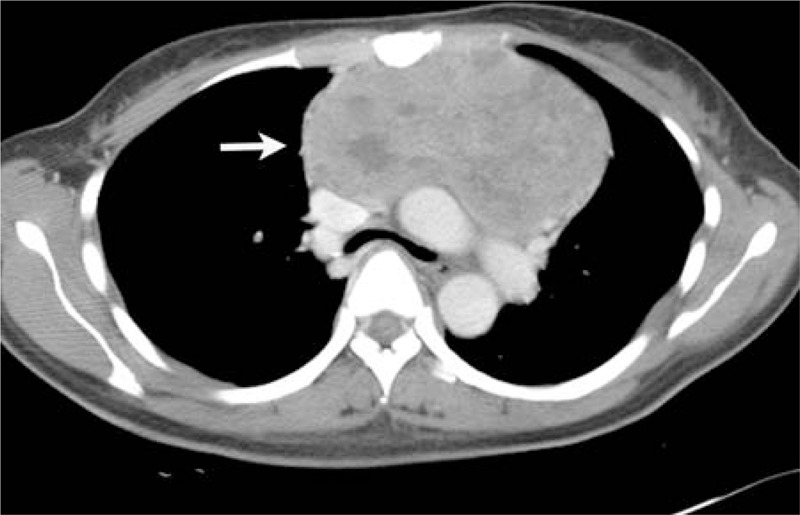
A 17-year-old man presented with a history of cough for about 2 months. Enhanced CT scan shows that the anterior middle mediastinal tumor had mild-to-intermediate heterogeneous enhancement, and adjacent large vessels were encased and displaced (arrow).
